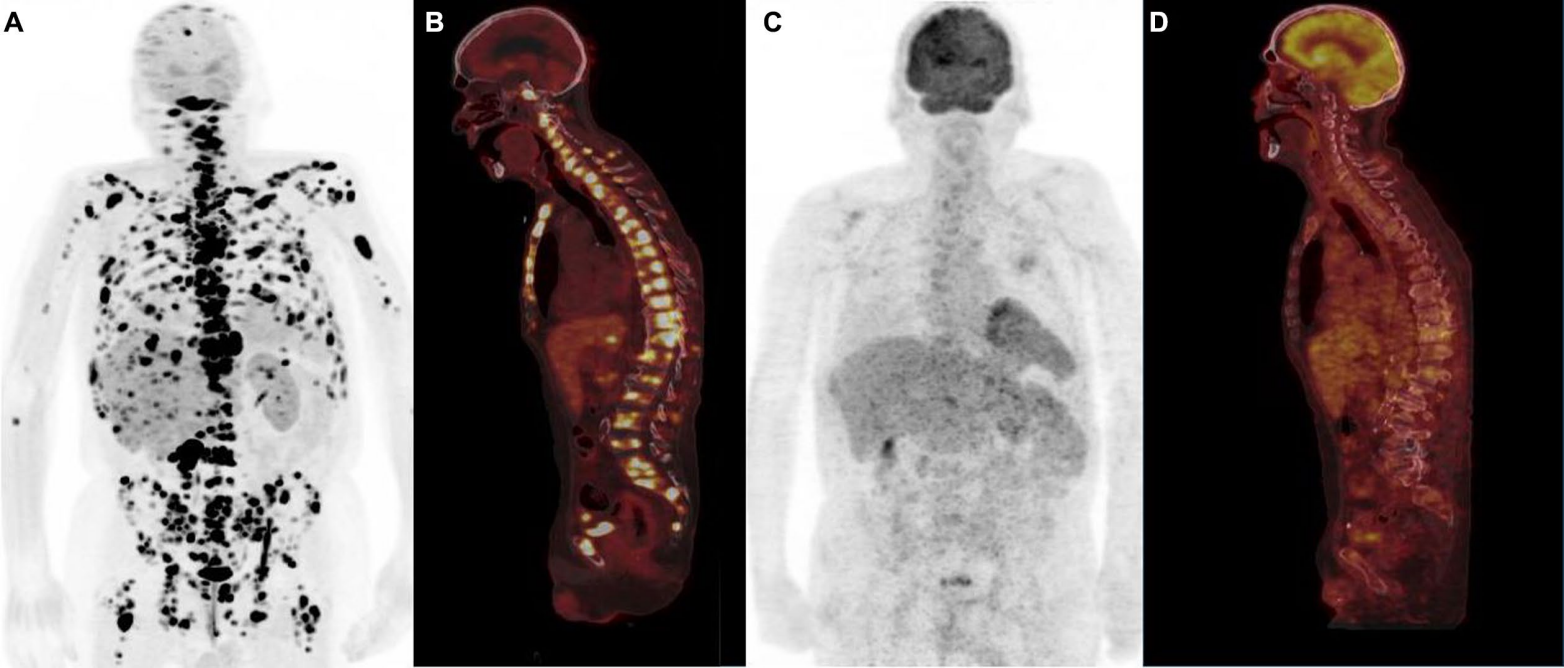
Typical example of “flip-flop” effect (anterior PET MIP, sagittal PET/CT) in a patient with metastatic low-grade (G1) small bowel NET. 18F-FDOPA PET showed multiple sclerotic bone metastases (A,B) not detectable by 18F-FDG PET (C,D), emphasizing the role of tumor grade in the selection of the optimal diagnostic radiotracer.
